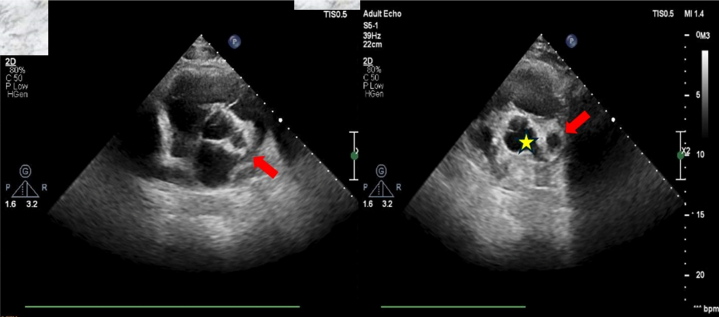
Parasternal Short-Axis View on Transthoracic Echocardiography Before Second Coronary Angiography Echolucent space (red arrows) next to the right coronary cusp of the aortic valve (star) suggests aneurysmal dilatation.
